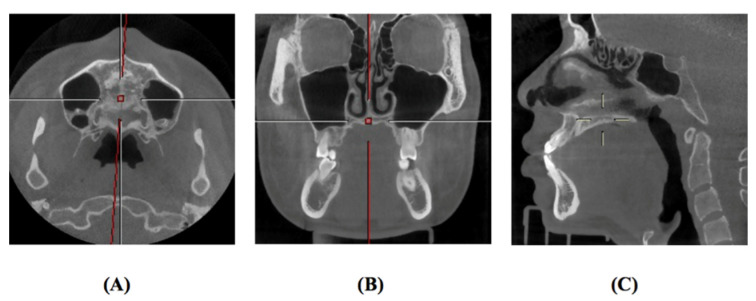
Head orientation in CBCT examination. (A): Axial view; (B): Coronal view; (C): Sagittal view.
